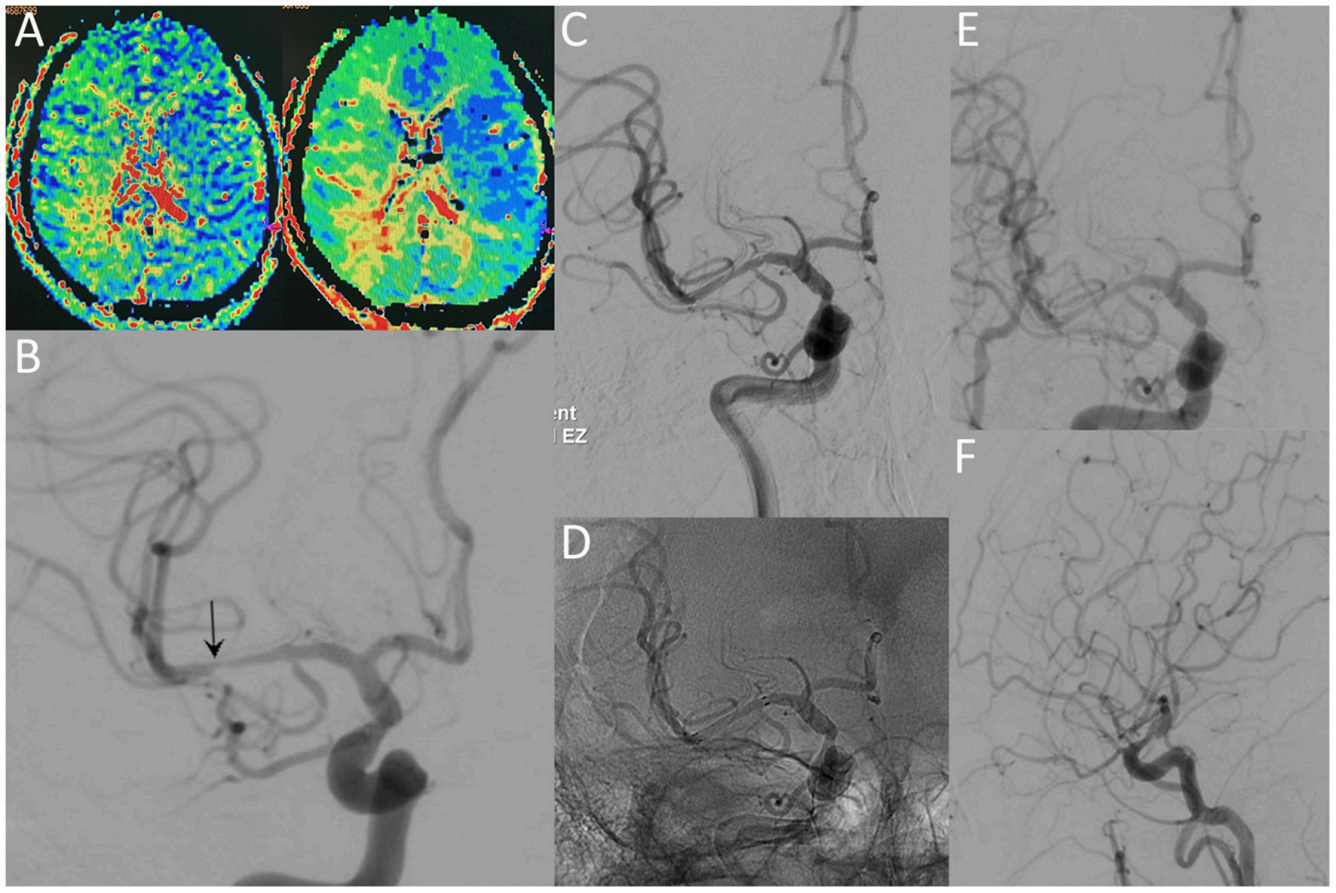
A 36-year-old male with a significant stenosis in the right MCA. CT perfusion images (A) reveal a prolongation of MTT and TTP before the procedure. Preprocedural DSA image (B) demonstrates high-grade stenosis close to the MCA bifurcation (arrow). Postprocedural DSA images (C,D) reveal patency of the MCA without residual stenosis. The 12-month follow-up DSA images (E,F) reveal no ISR in the region of the originally stented lesion.
